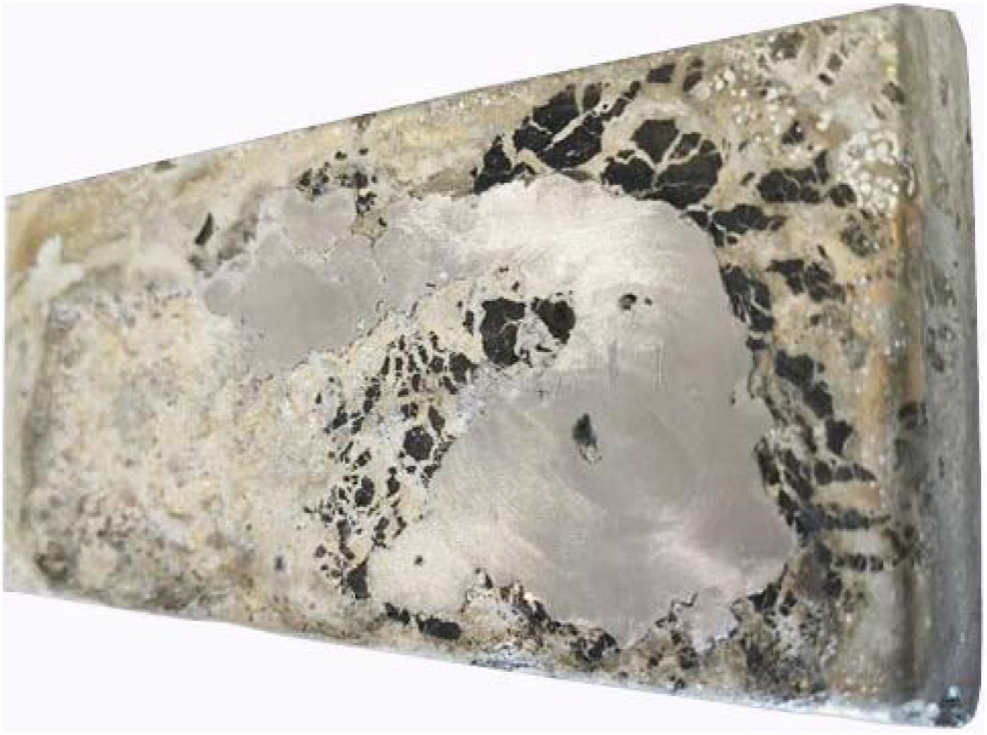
Ingot after ultrasonic cleaning.
